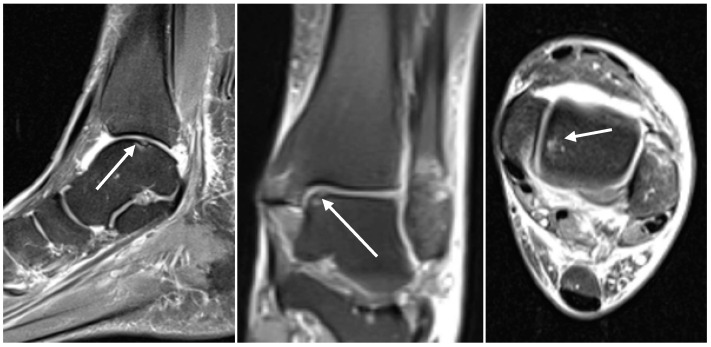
Sagittal (left), coronal (middle), and axial (right) proton-density-weighted turbo-spin-echo fat-saturation MRI sequences (PD tse fs) showing CLs (white arrows) of the talar dome in a 59-year-old female with an acute ankle fracture.
